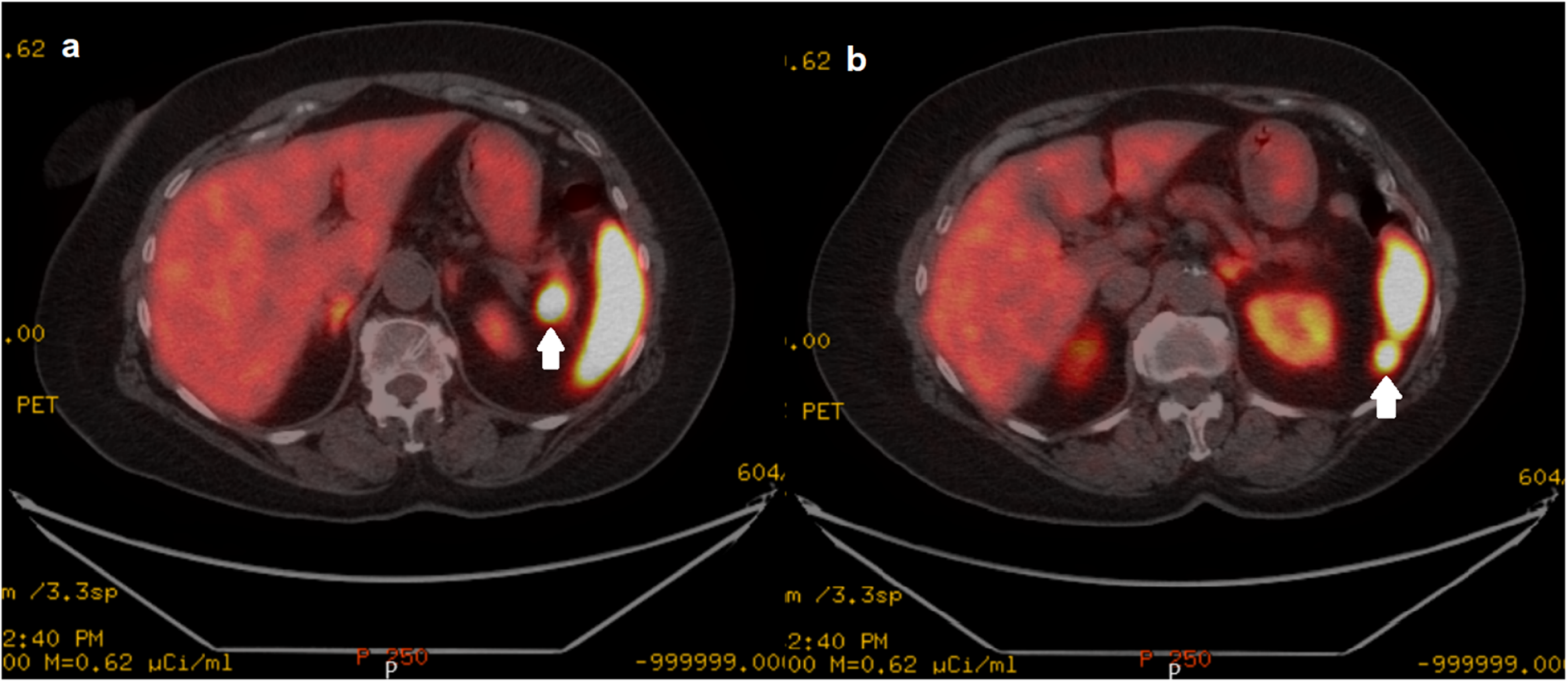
68Ga-DOTATATE PET/CT images, revealing two masses with intense tracer uptake in the pancreatic tail/splenic hilum (a) and in the inferior pole of the spleen (b)
